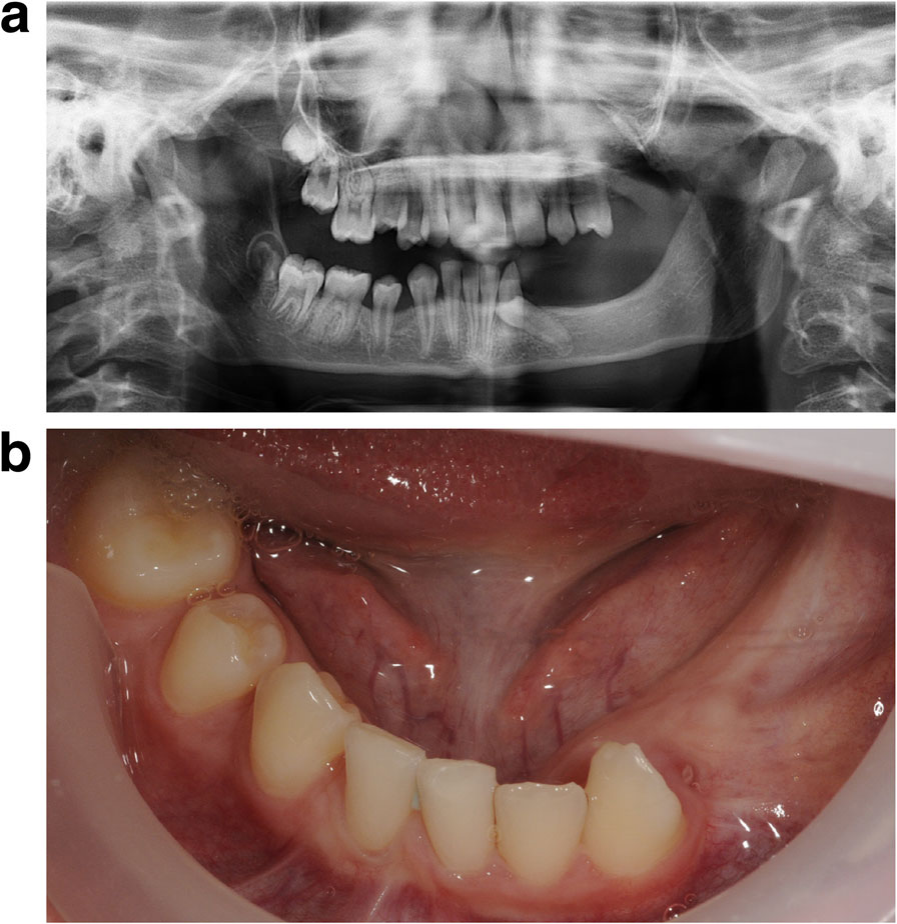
a Patient 2—pre-implant orthopantomogram (OPG) at the age of 12. Situation before start of orthodontic and implant treatment. Eleven permanent teeth (including 2 third molars) were congenitally missing and the 34 is impacted. To erect the 34, orthodontic treatment was desired. Due to the lack of stable anchorages in the third quadrant, it was decided to place one implant at tooth region 35 for orthodontic anchorage and future prosthetics. Due to very limited bone height virtual implant planning was needed to avoid damage to the mandibular nerve. b Patient 2—mandible, pre implant intra-oral situation at the age of 12. The 34 is not visible in the oral cavity
